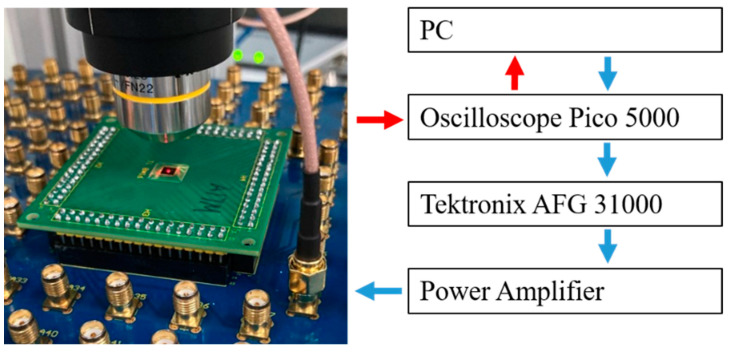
Laser Doppler vibrometer (LDV) setup for characterizing the PMUT performance.
